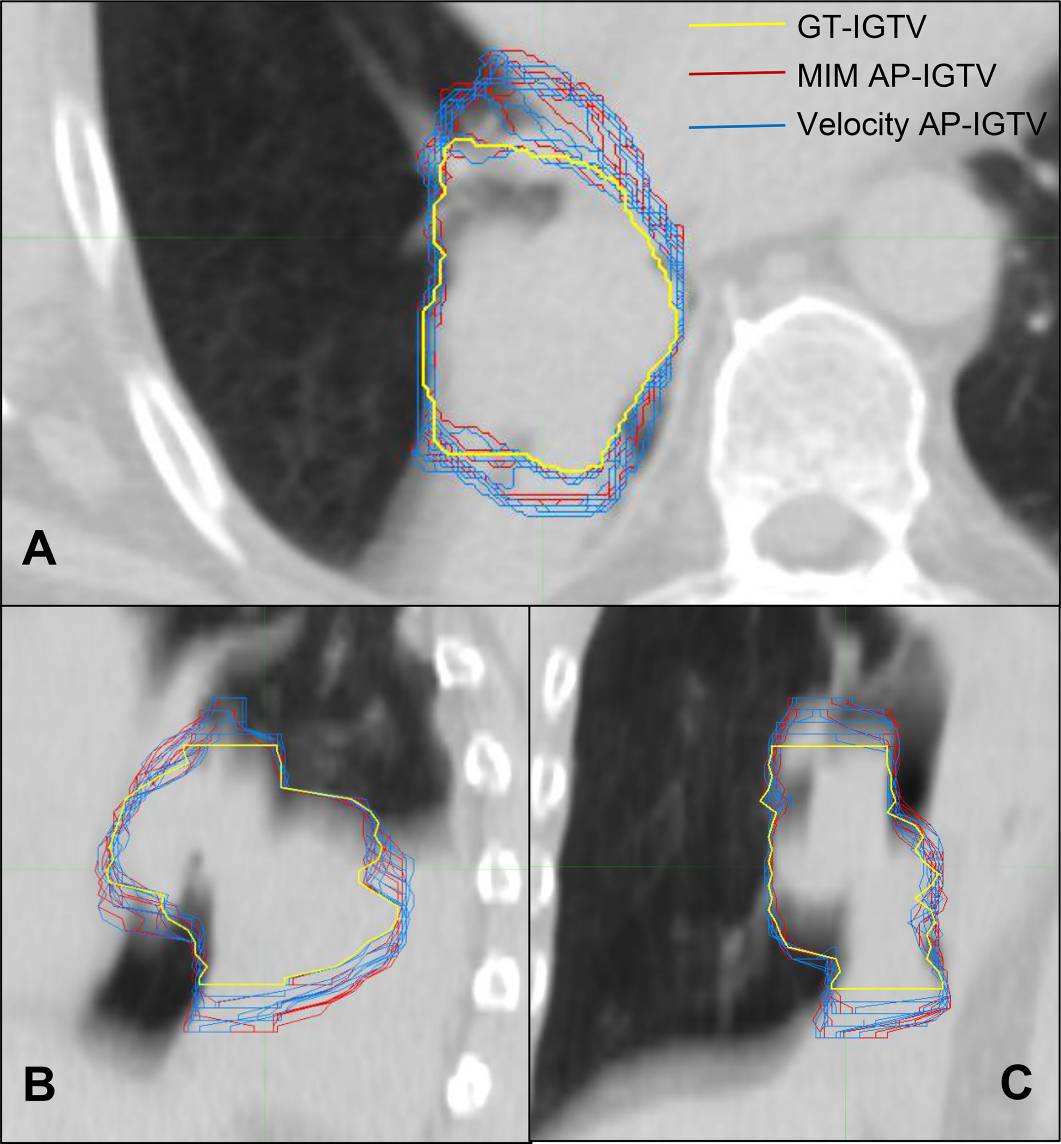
CT cross sections of one locally advanced non-small cell lung cancer (LA-NSCLC) lesion representative of the study cohort in axial (A), sagittal (B), and coronal (C) planes with variation in auto-propagated internal gross tumor volumes (AP-IGTV) derived from the two employed deformable image registration (DIR) algorithms—MIM (outlined in red) and Velocity (outlined in blue)—compared to the ground-truth internal gross tumor volume (GT-IGTV; outlined in thick yellow).
